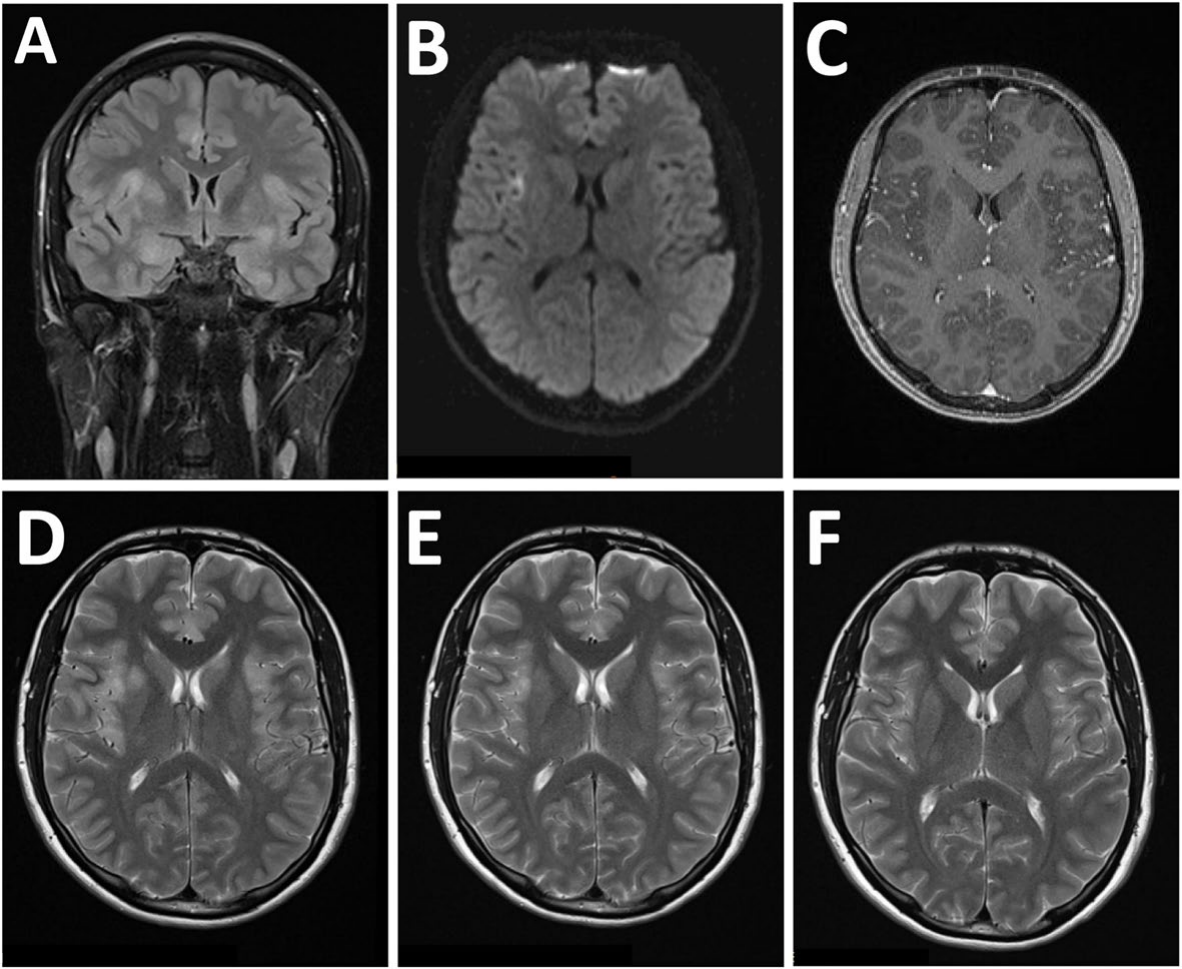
Serial MRI examinations. A-D, at initial examination (d 11 after PCR diagnosis), FLAIR (A) showed increased signal intensity bilaterally, in the cortex and basal ganglia. B DWI showed only a small area of restricted diffusion in the right insular cortex. C There was no signal enhancement after i.v. gadolinium. D-F T2 images showing signal hyperintensities before (d 11) (D) and improvement after 5 days of i.v. methylprednisolone (d17; E). F Full resolution (d 35)
